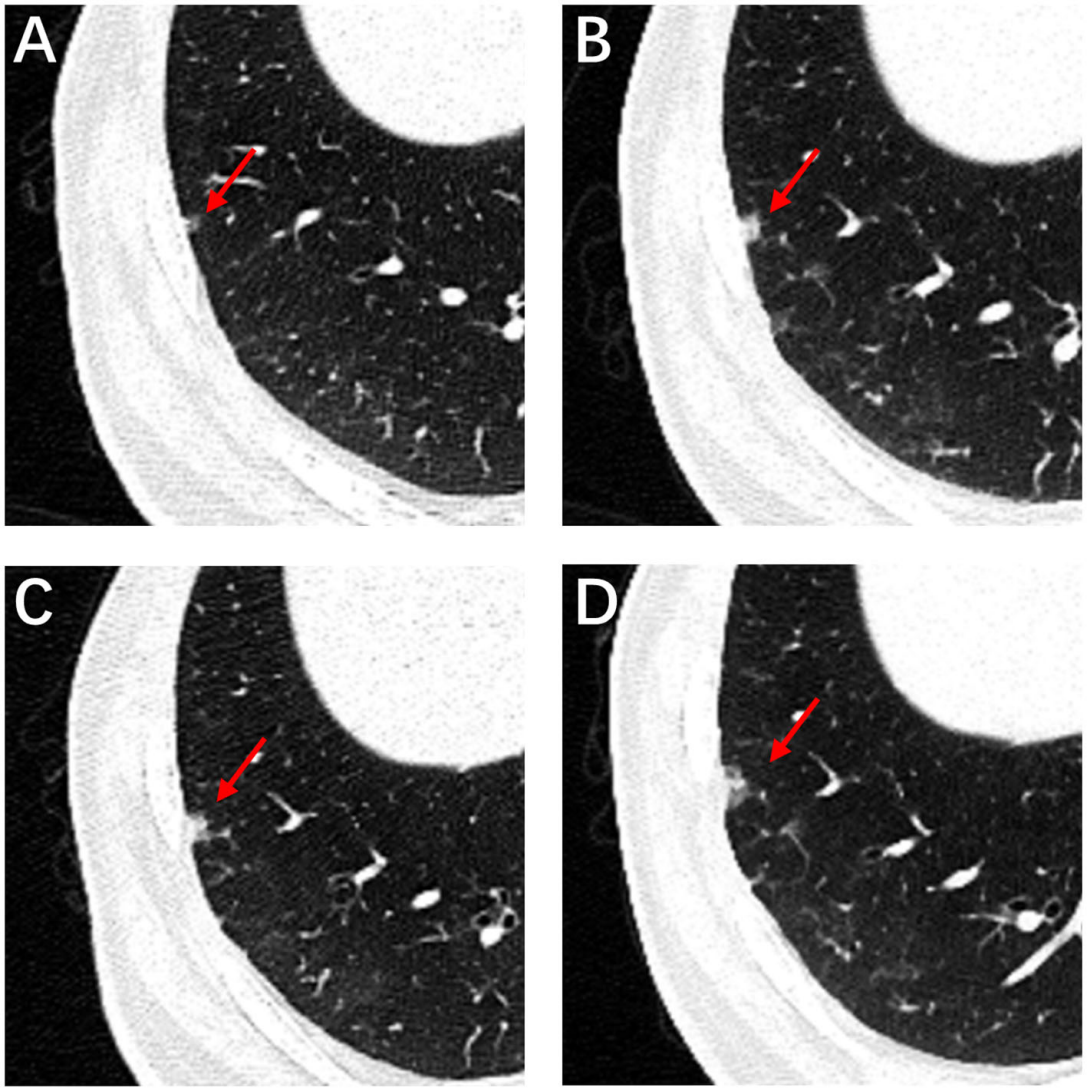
High-resolution computed tomography. CT image of the patient with subsolid nodule changes during 4 years. Initial CT image shows a subsolid nodule in the right lower lobe subpleural areas measured approximately 4 mm, with a round shape, smooth margin and clear tumor-lung interface (A, red arrow). CT imaging data from physical examination from 2018 to 2020 (B–D, red arrow).
